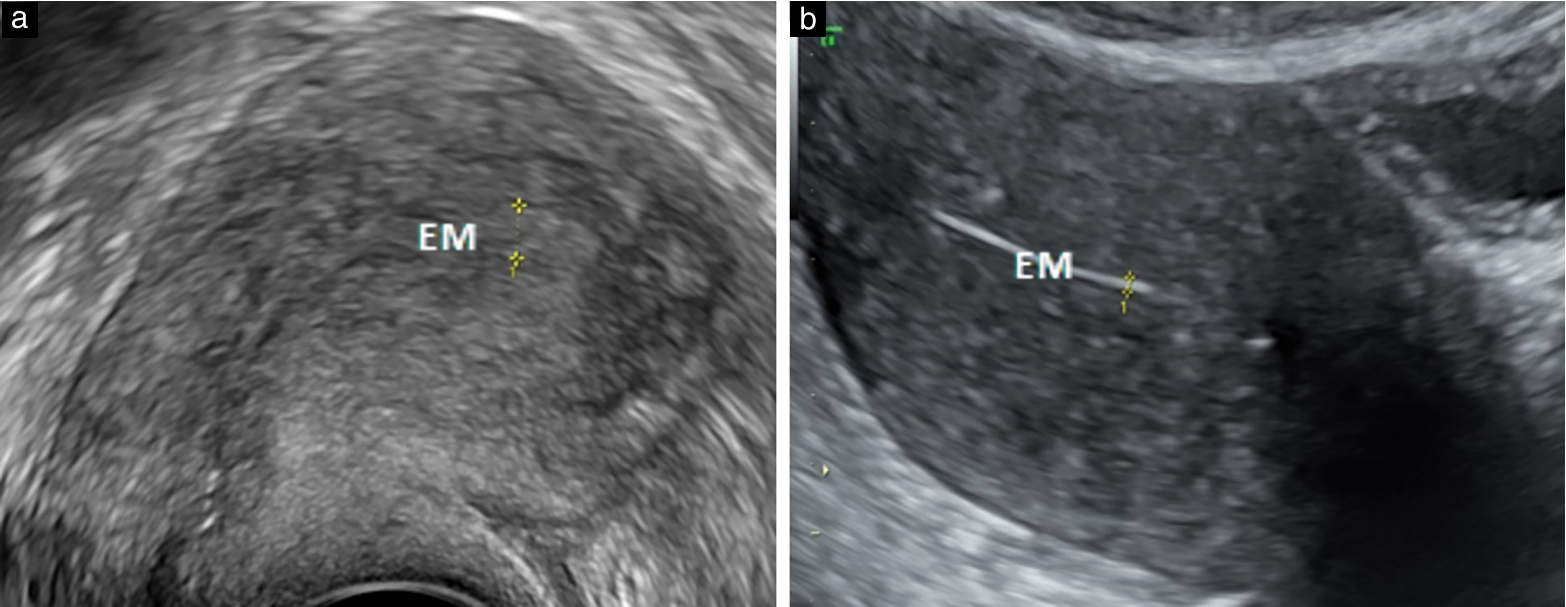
Visualization of endometrium (EM) in the presence of adenomyosis on transvaginal ultrasound (a) and on transabdominal ultrasound (multimodal approach) (b). Calipers indicate EM thickness.
